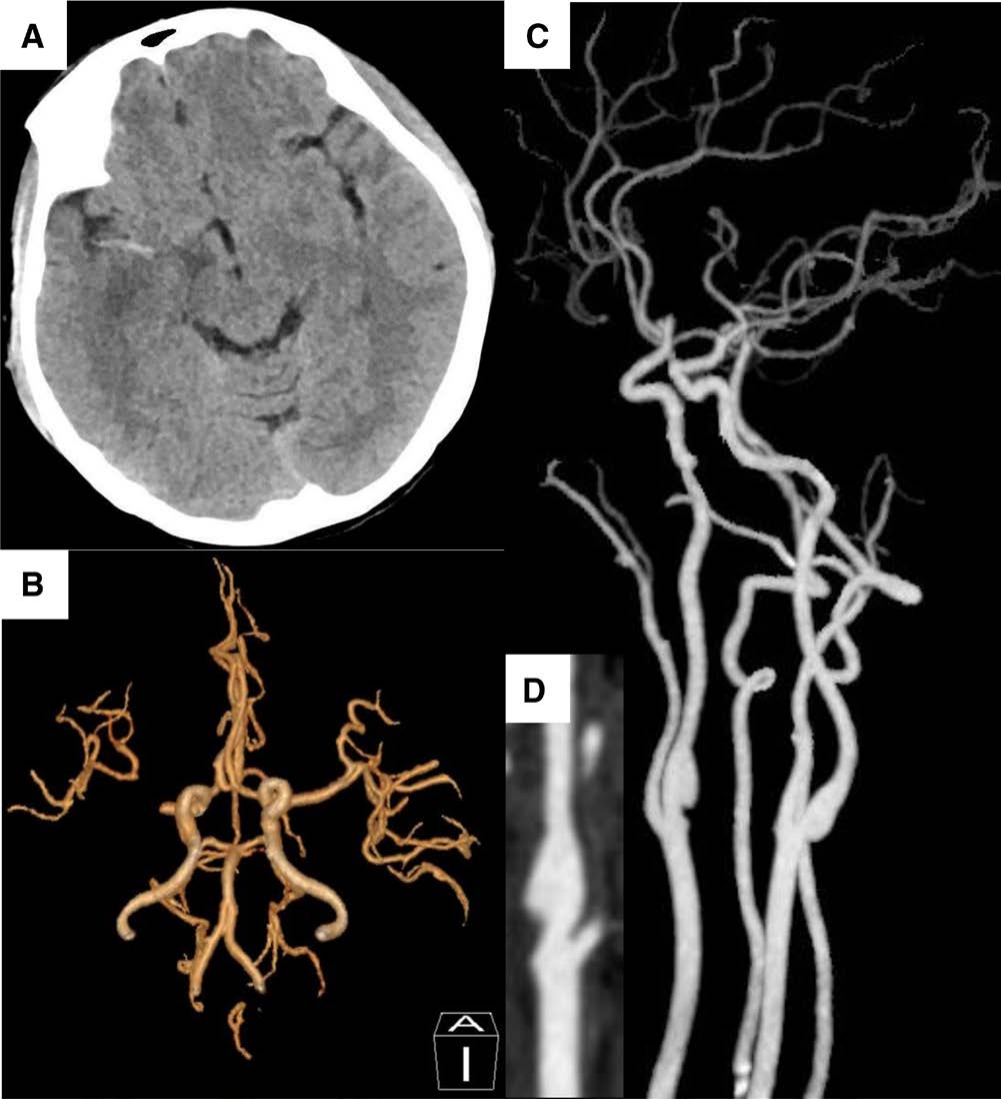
(A) The reexamination of brain CT showed a hyperdense MCA sign and (B) computed tomographic angiography showed M1 of right middle cerebral artery re-occluded and (C and D) the thrombus superimposed to the web. CT = computed tomography, MCA = middle cerebral artery.
